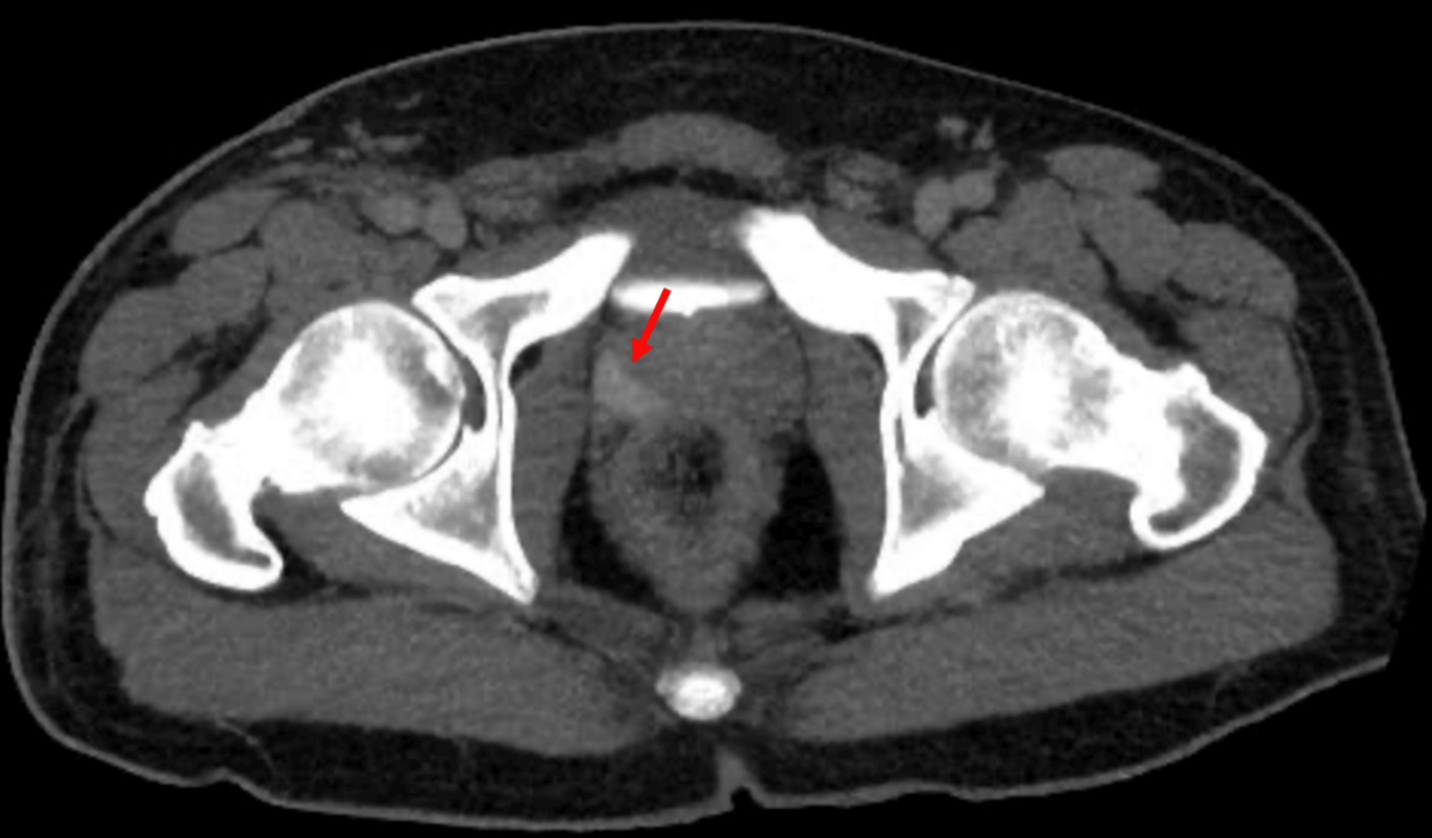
CT of the abdomen and pelvis. The red arrow points to the right-sided peripheral zone hyperdensity in the prostate.
